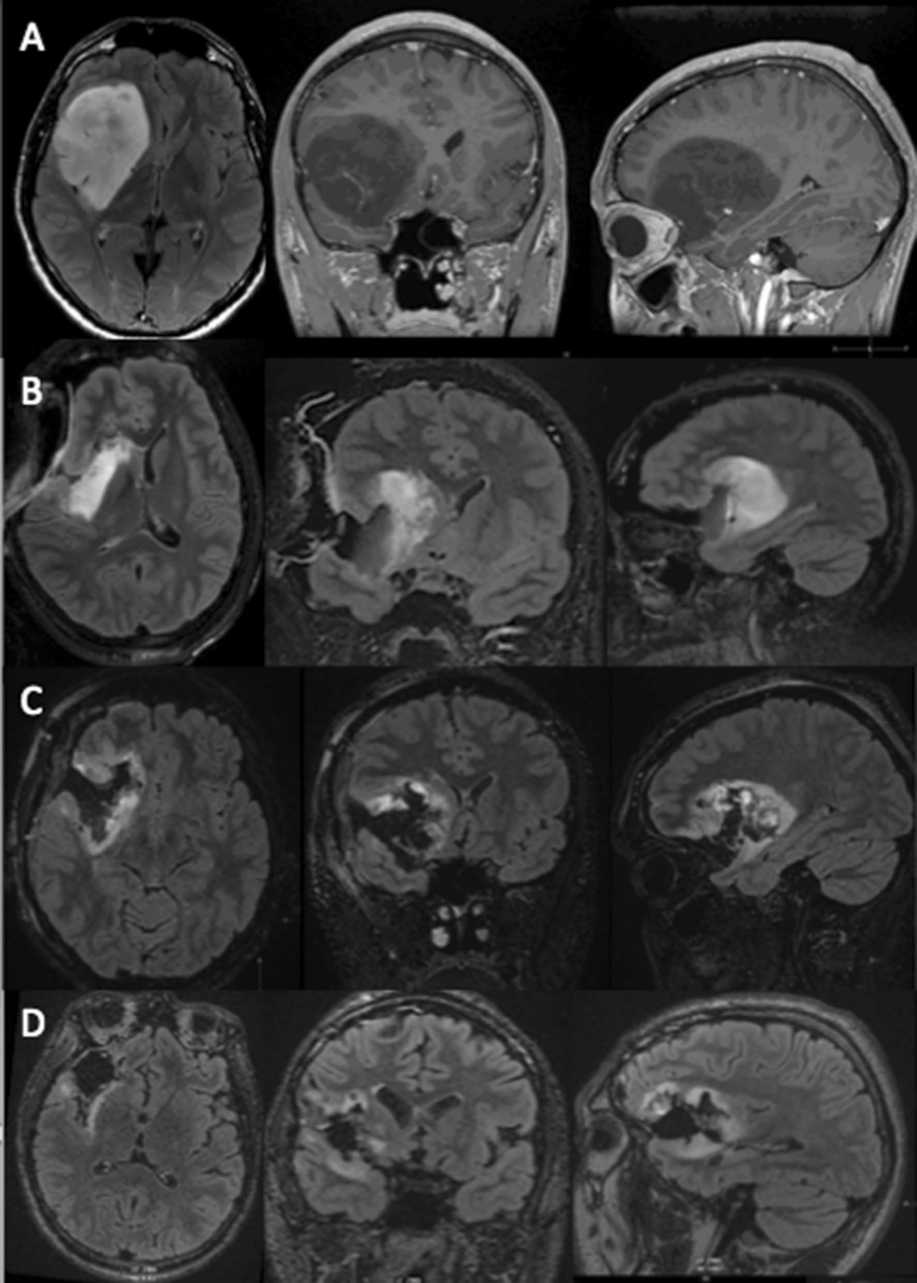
Staged surgical approach for a right fronto-temporo-insular glioma. (A) Preoperative MRI depicting a hypo signal T1 lesion, with sharp contours. The preoperative volume was of 112 cm^3^. (B) 1st IoMRI reporting a tumor remnant at the inner and posterior part of the insula and in the frontal lobe of 38 cm^3^. Additional resection was performed. (C) 2nd IoMRI, the tumor residue was of 11 cm^3^. Due to the presence of the internal capsule at the superior part of the lesion the end of surgery was decided. (D) Postoperative control at 3 months, with less perioperative signal abnormalities the residue was reassessed, with a volume of 6 cm^3^, corresponding to an EOR of 95% (using IntelliSpace Portal, Philips, module “tumor tracking.” version 9.0 (www.philips.fr).
